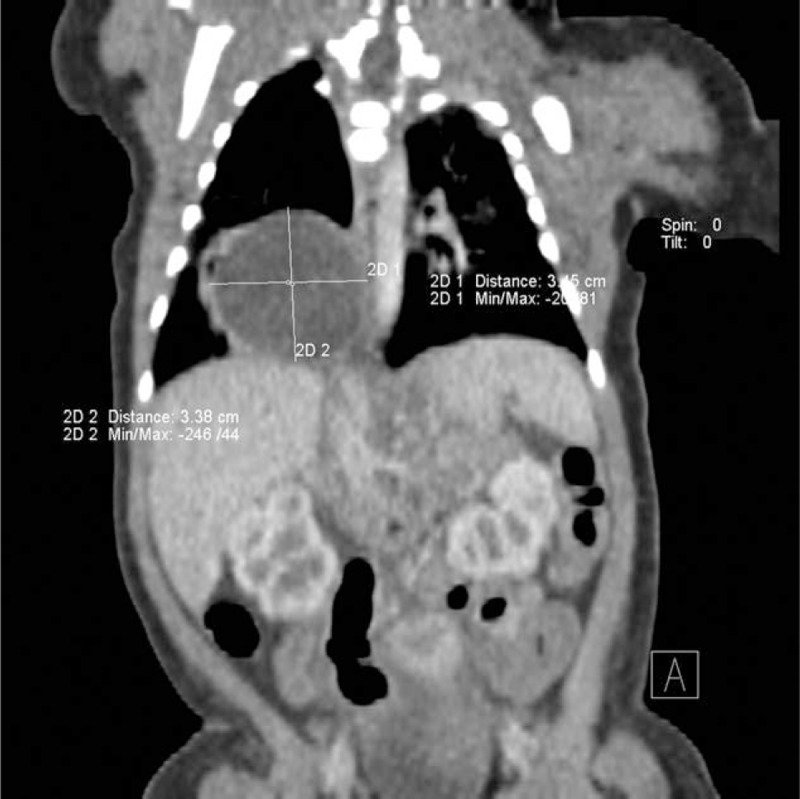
Computed tomography scan. Esophageal duplication cyst with the size fixed (frontal view).
